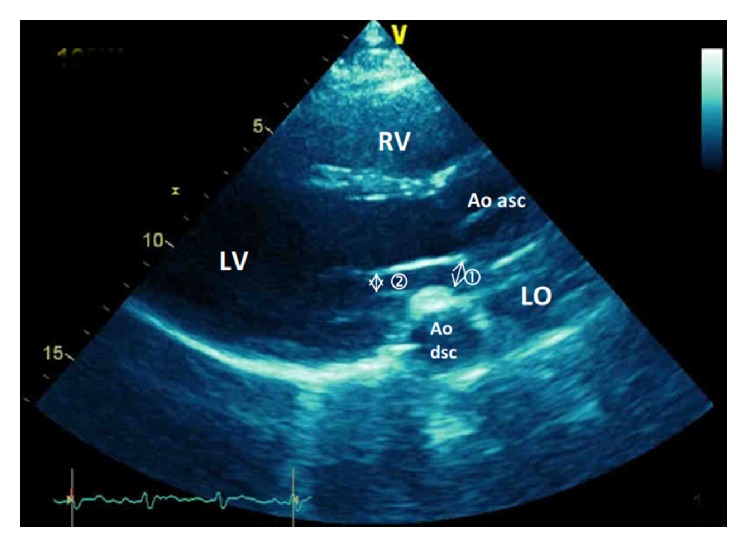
Echocardiography at maximal exercise. RV, right ventricle; LV, left ventricle; LO, left atrium; Ao dsc, descending aorta; Ao asc, ascending aorta; ①, compression of the mitral annulus; ②, compression of the mitral orifice.
